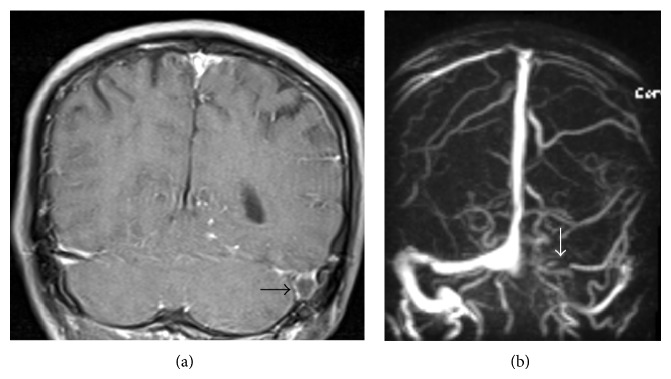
Dural venous sinus thrombosis as an only imaging evidence of tuberculous meningitis in a 45-year-old male who presented with headache and cerebrospinal fluid PCR positive for* Mycobacterium tuberculosis*. (a) Coronal postcontrast T1-weighted MR image demonstrates a filing defect within dilated left sigmoid sinus (*black arrow*). (b) MR angiogram reveals nonvisualization of transverse and sigmoid sinuses in left side (*white arrow*).
